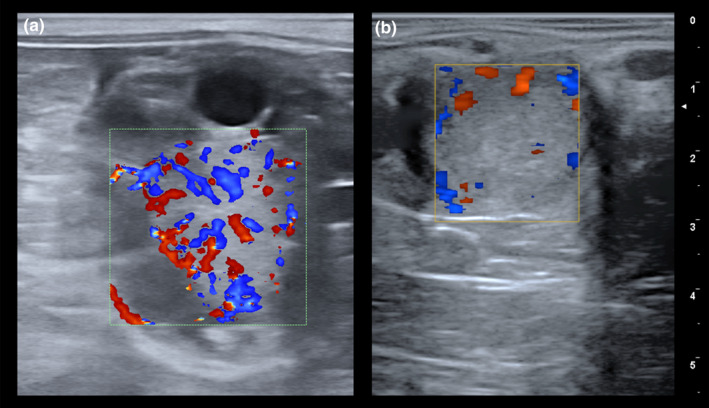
Colour Doppler evaluation of the corpus luteum (CL) in the mare. (a) Colour Doppler ultrasound image of a CL displaying a well‐established homogeneous pattern of vascularization, consistent with an active status of this gland. (b) Colour Doppler ultrasound image of a CL showing an absence of irrigation, indicative of a low functionality
